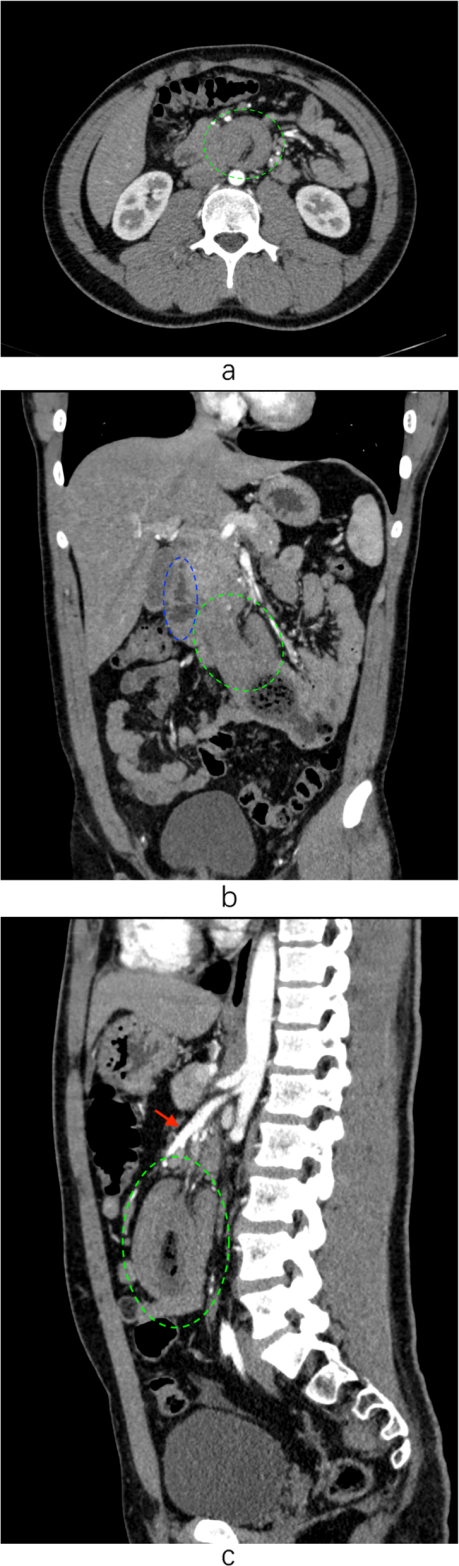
a. The cross section of abdominal CT shows the typical bowel-within-bowel sign suggestive of duodenal intussusception into the jejunum (green circle). The intestinal wall of the head was thickened. The degree of strengthening was slightly reduced. Lumpy contents can be seen in the duodenal cavity. b. The coronal section of the CT scan shows the intussusception of duodenum into the jejunum (green circle) and the descending part of duodenum (blue circle) c. The sagittal section of the CT scan shows the intussusception (green circle) and its positional relation with superior mesenteric artery (red arrow)
